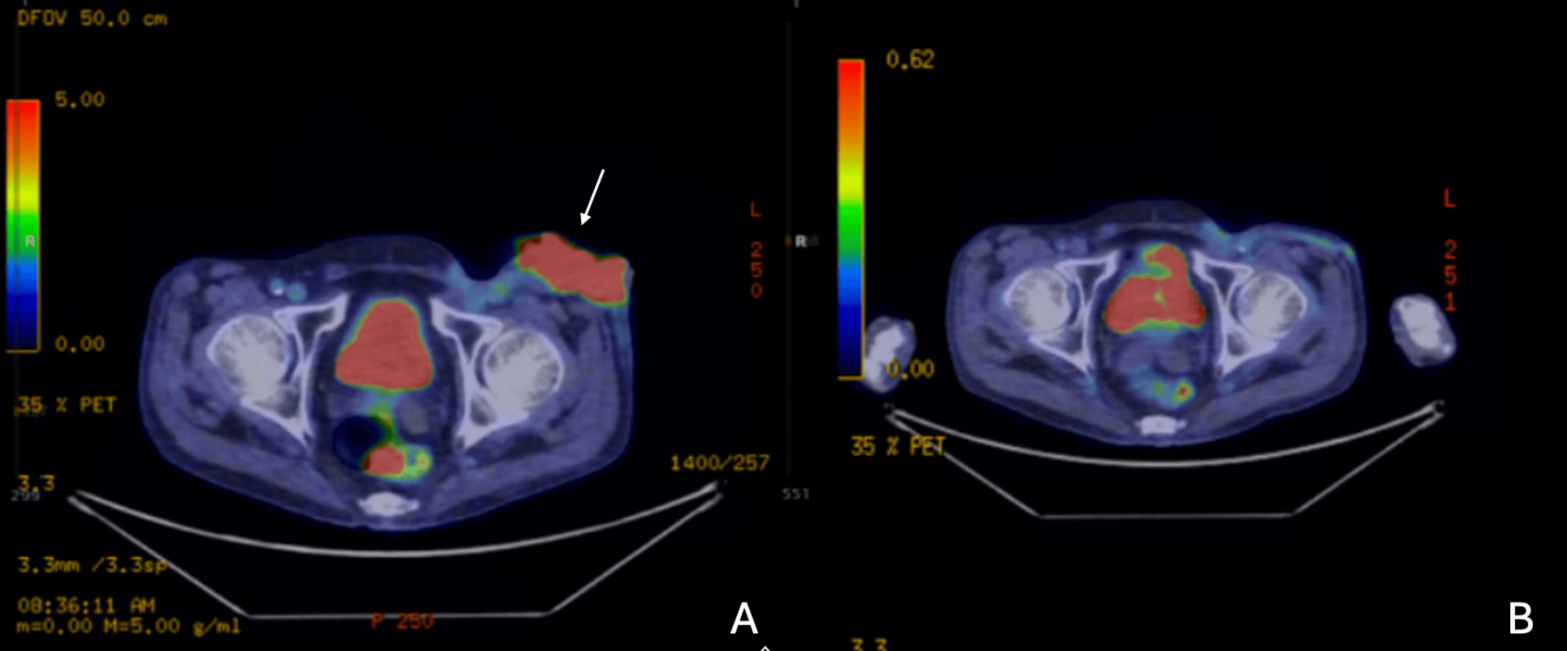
PET-CT imaging before resection (A) demonstrating left thigh mass (white arrow) and six weeks after surgical resection (B). Axial view PET/CT fused image. Image A demonstrates pre-resection of left posterior upper thigh soft tissue mass with SUV of 11.2 measuring 8x3cm, and image B demonstrates post-resection without evidence of lymphoma.
